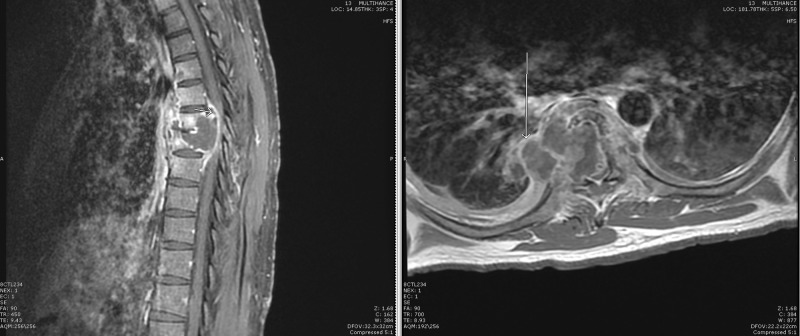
MRI thoracic spine Sagittal (left) and axial (right) views of contrast-enhanced T1-weighted magnetic resonance imaging (MRI) showing abnormal signal with loss of vertebral body height and mild retrolisthesis at T6 and T7. There is also a peripherally enhancing collection within the T6-7 disc space extending into the paraspinal soft tissues and epidural space, causing cord compression. Subligamentous spread is seen anteriorly from the T4 through T8 levels.
